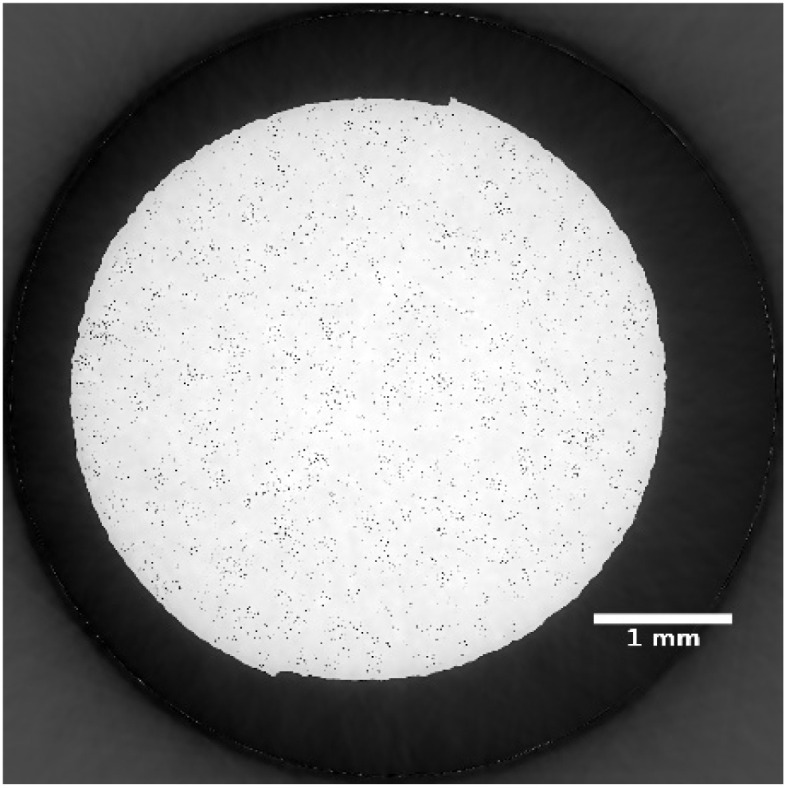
Cross section slice of sample 5 obtained using X-ray microtomography.
